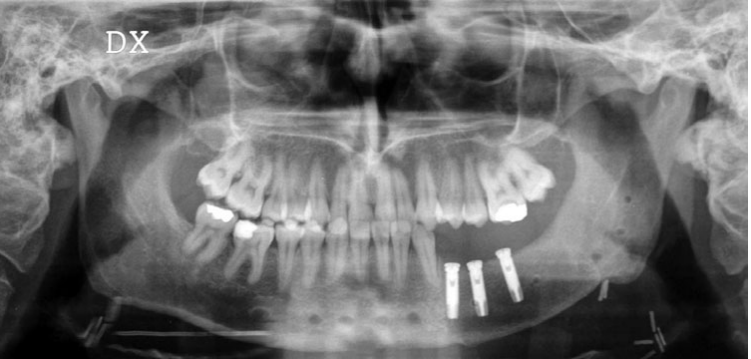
panoramic radiograph of patient n°1, six months after surgery, and after the plate removal and implants insertion.
